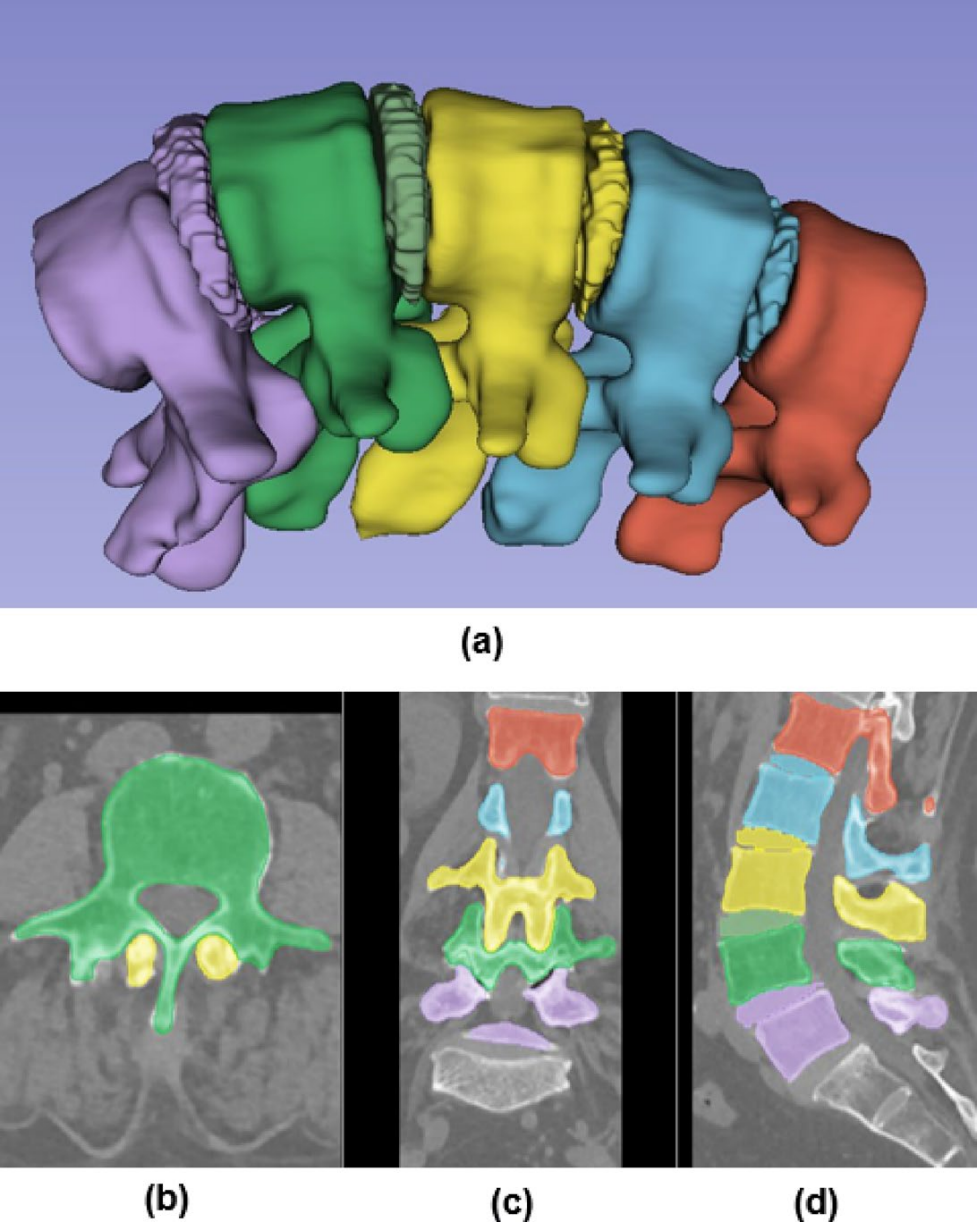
Automated segmentation and 3D visualization of the lumbar spine. (a) Reconstructed 3D model with color-coded vertebrae, intervertebral discs, and associated structures. (b) Axial view of the segmented CT image, showing vertebrae and intervertebral discs. (c) Coronal view with labeled vertebrae and intervertebral discs. (d) Sagittal view of the segmented spine, processed using nnUNet for segmentation and MONAI for labeling, color mapping, and refinement.
